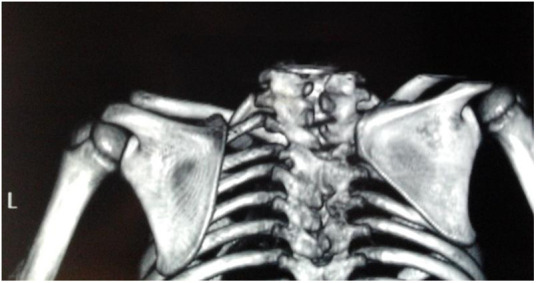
Postoperative 3D-CT confirmed successful descent of the right scapula to a level symmetric with the contralateral side, along with resection of the inferior angle.
